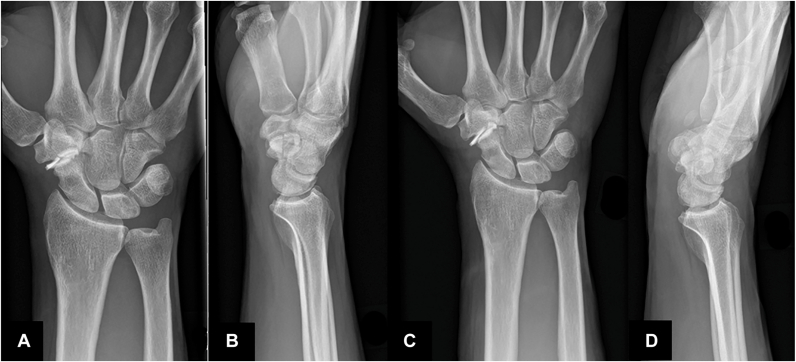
X-rays of implant failure. A, B First failure. C, D Second failure.
